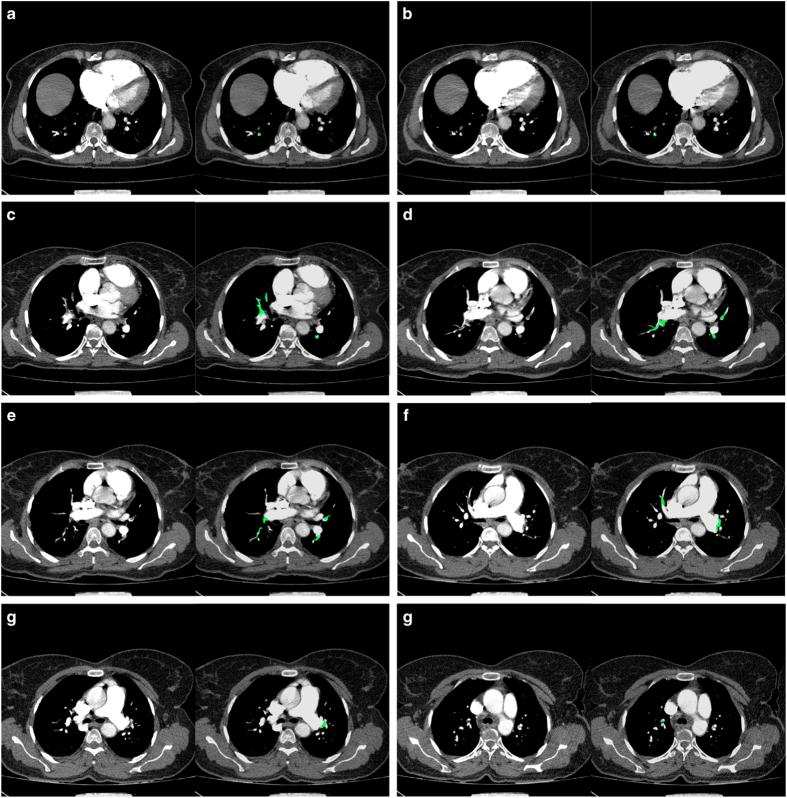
A sample CTA image of FUMPE. Including the source (left-hand side in each pair) and ground-truth (right-hand side in the same pair) images corresponding to the (a) 77th, (b) 80th, (c) 106th, (d) 116th, (e) 119th, (f) 133th, (g) 139th, and (h) 151th slices of Patient16.
